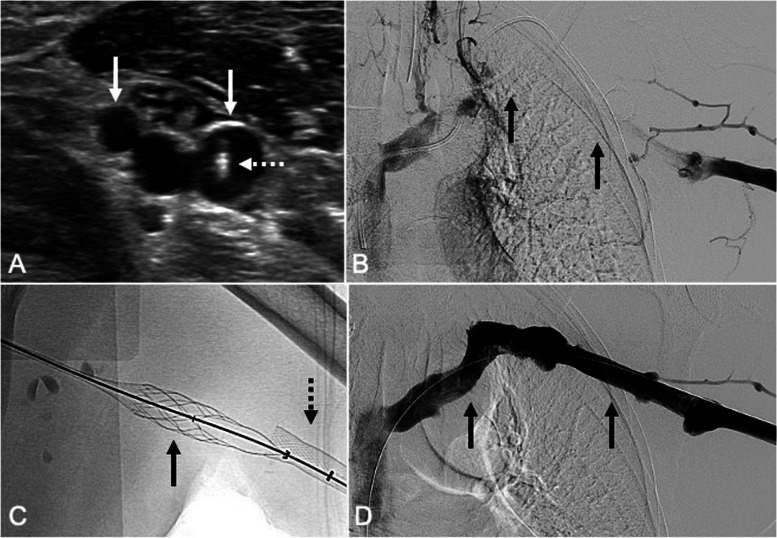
A 54-year-old man with breast cancer post right mastectomy undergoing chemotherapy via a left-sided chest port presented with left arm swelling after playing tennis. A Ultrasound-guided access of one of the paired brachial veins (solid arrows) was obtained using a 21-gauge micropuncture needle (dashed arrow). B Left upper extremity venography demonstrated occlusion of the left brachiocephalic, subclavian, and axillary veins (solid arrows). C Thrombectomy of the left upper extremity veins was performed with the 13.5-French ClotTriever Thrombectomy System (solid arrow) after upsizing the access sheath to the funnel-tipped ClotTriever Sheath (dashed arrow). D Completion left upper extremity venography demonstrated brisk in-line flow from the left brachial vein to the right atrium (solid arrows)
